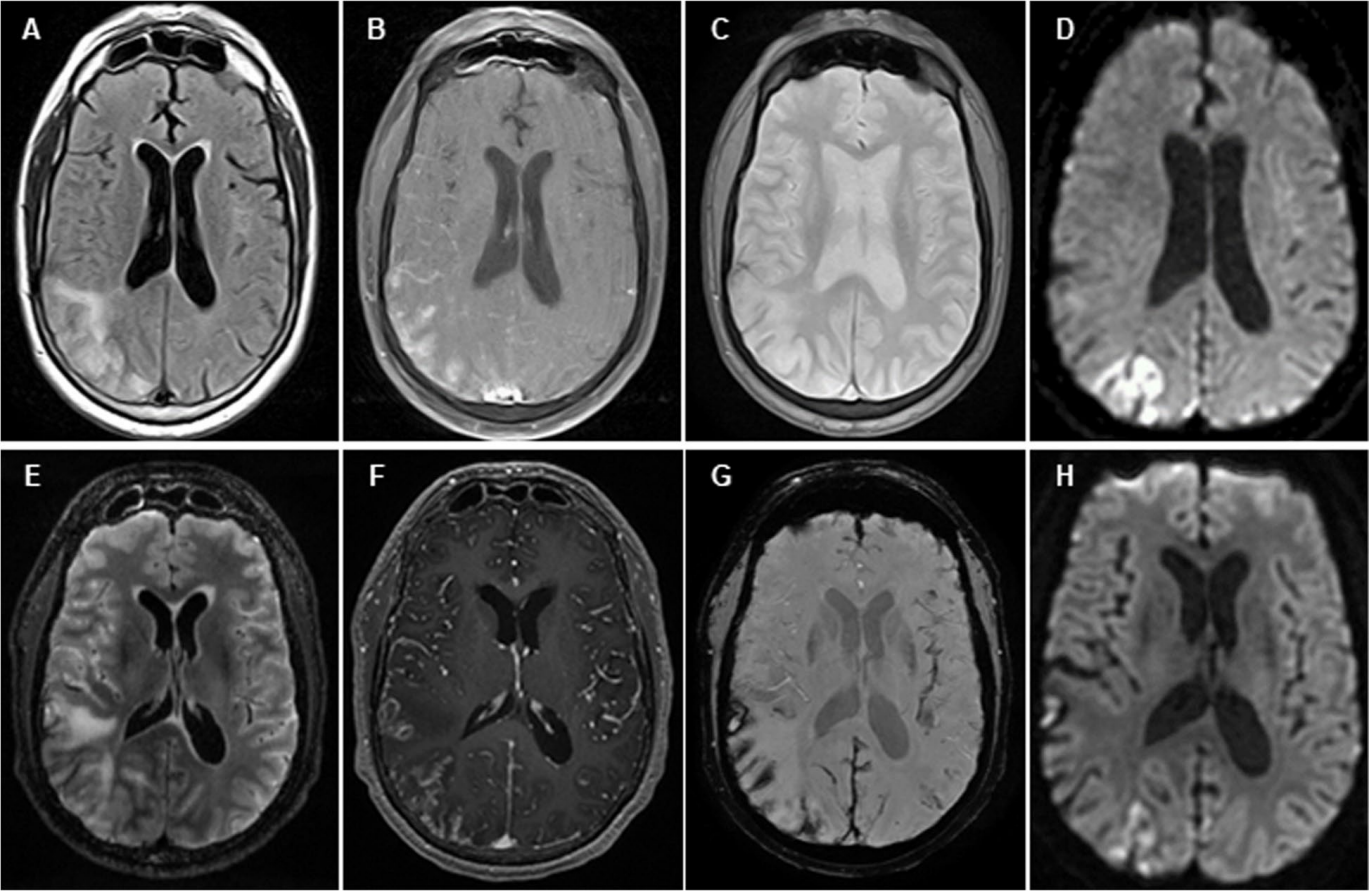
MRI Studies. Top row: MRI obtained on hospital re-presentation, 1 month after symptom onset. There is co-localized right temporo-parieto-occipital T2 hyperintensity with vasogenic edema (A), leptomeningeal enhancement on T1 post-contrast (B), and cortical superficial siderosis on T2 gradient echo sequences (C). Multifocal right parieto-occipital (D) and bilateral cerebellar punctate (not shown) infarcts were present on diffusion-weighted imaging. Bottom Row: MRI obtained on hospital transfer 1 week later and prior to first brain biopsy. There is T2 hyperintensity with vasogenic edema in the right temporo-parietal lobe and FLAIR non-suppression (E) with associated diffuse leptomeningeal enhancement on T1 post-contrast (F) and diffuse cortical superficial siderosis on susceptibility weighted imaging (G). Multifocal right parieto-occipital (H) and new left frontal punctate (not shown) infarcts were present on diffusion-weighted imaging
